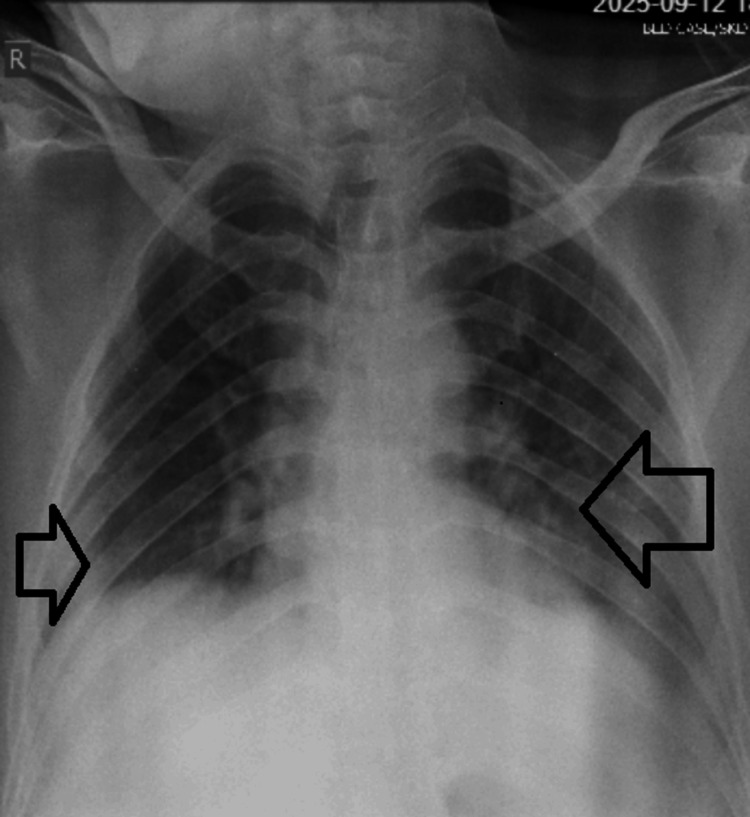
Bedside chest radiograph on Day 1 The image shows patchy, ill-defined opacity in the right lower zone adjacent to the diaphragm and another ill-defined left perihilar haziness spreading to the mid-lower zone, showing bilateral pneumonia (arrows)
